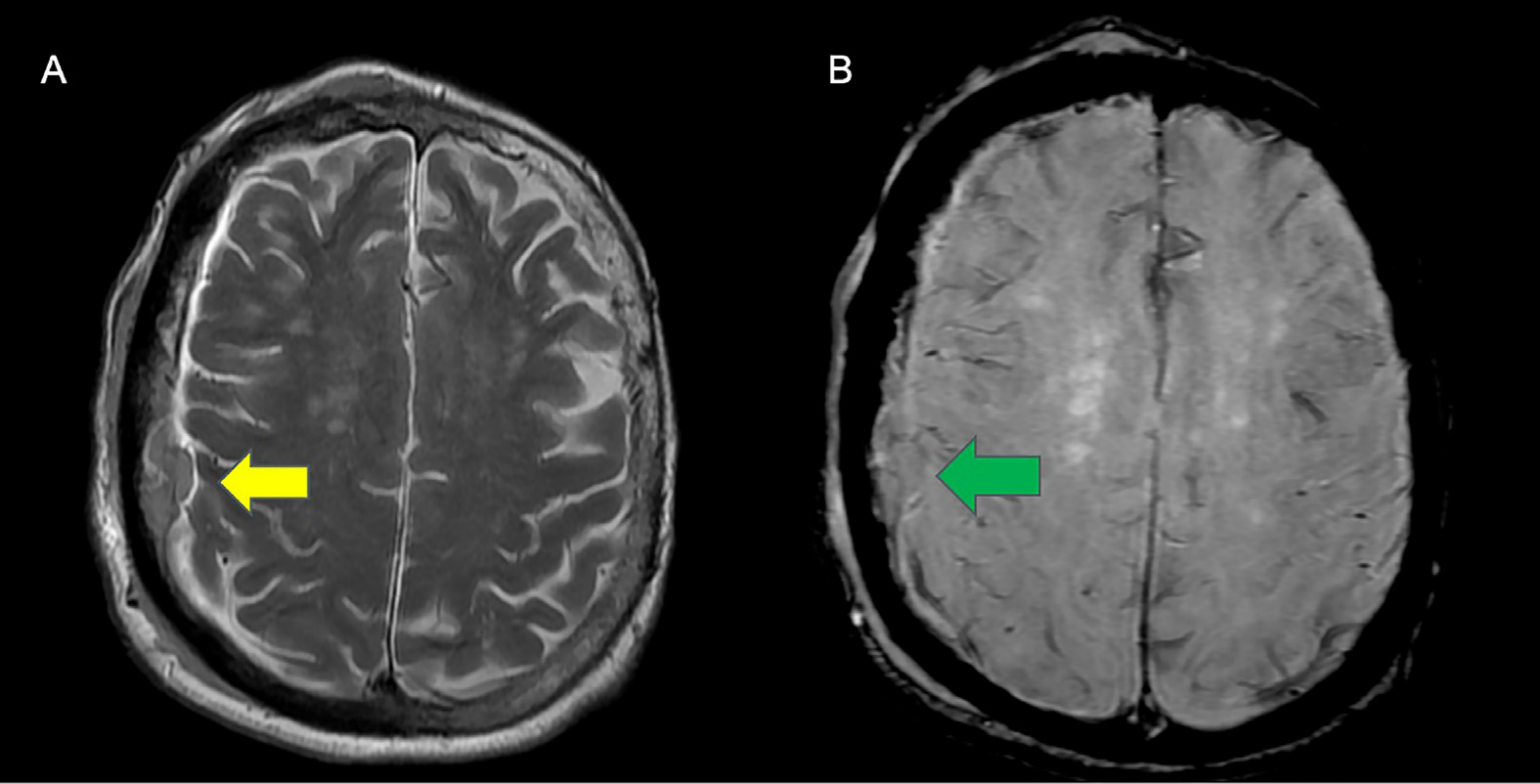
Magnetic resonance imaging of the brain, axial views. (A) T2 sequence showing a hypointense right sided subdural collection (yellow arrow). (B) SWI sequence showing no blood products withing the right sided subdural collection (green arrow).
